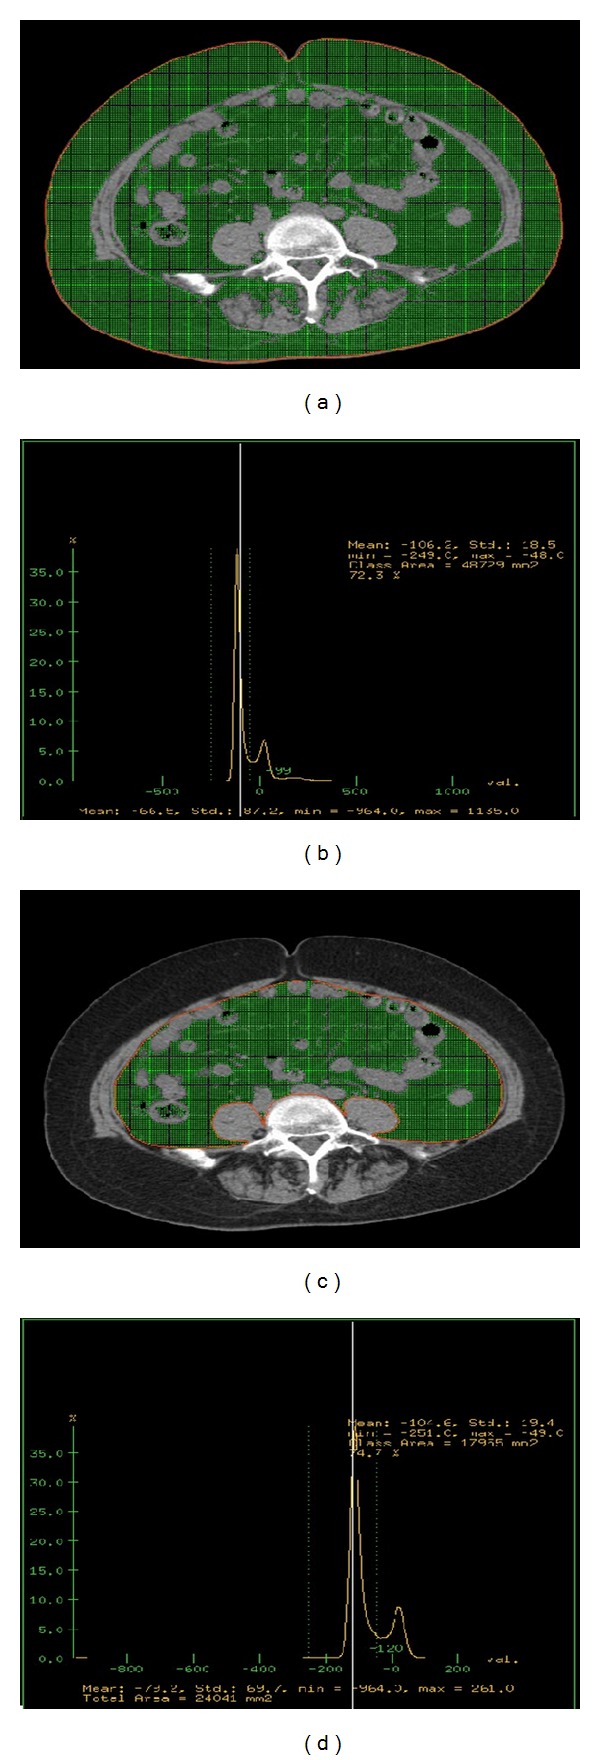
(a)–(d) are the measurement for abdominal fat. (a) is the line along abdominal skin at the axial position of CT image (red line); (b) is the area within adipose tissue of this area with histogram calculation (green area), that is, the total abdominal adipose tissue (TA) (mm^2^); (c) is the line along the abdominal wall and the internal edges of bilateral psoas major (red line); (d) is the area of adipose tissue within this area with histogram calculation (green area), the visceral adipose tissue (VA) (mm^2^).
